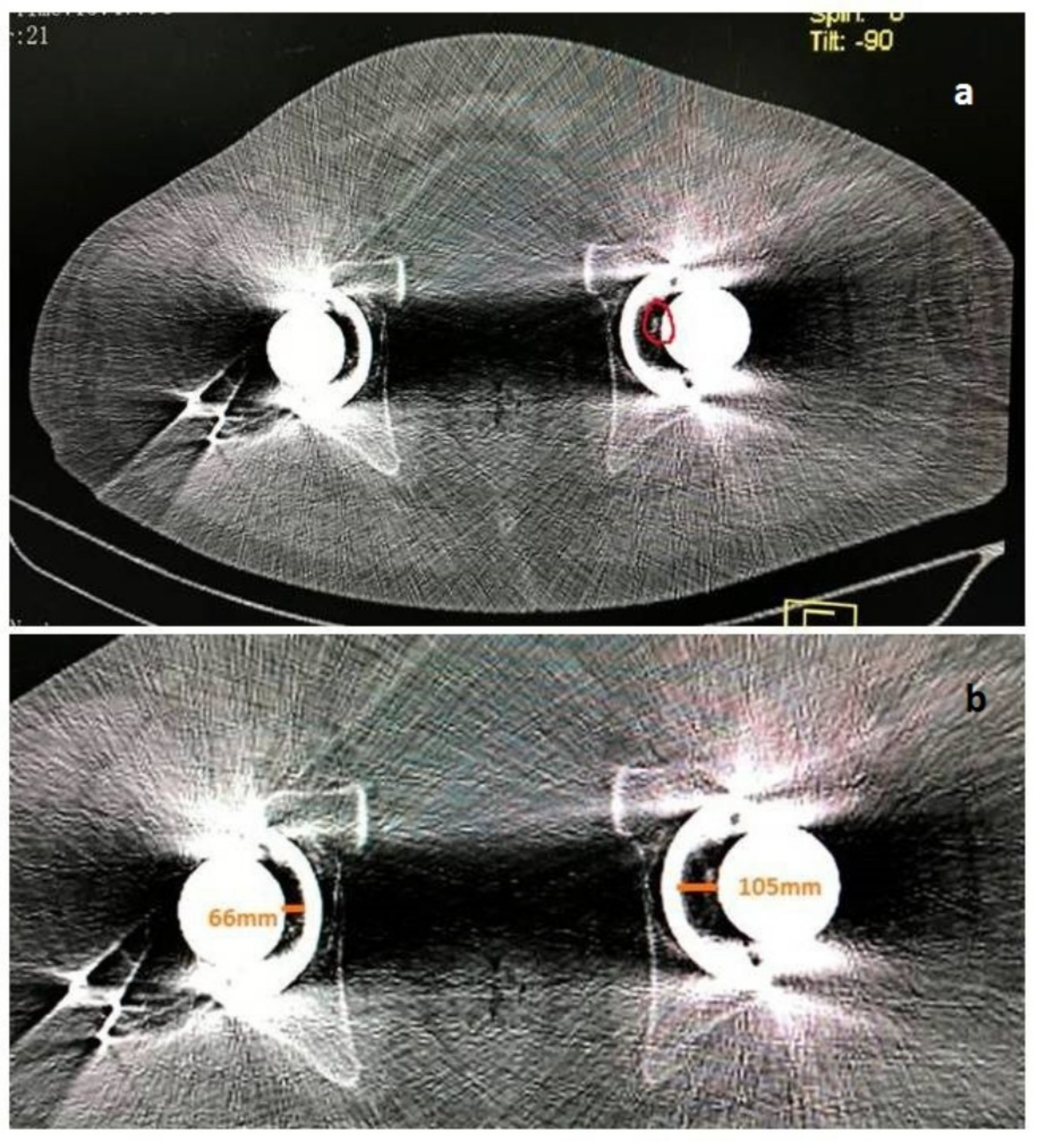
Axial section on computed tomography. a) The loose bone piece (red encirclage). b) The distance from the center of the acetabular shell to that of the femoral head is also increased.
